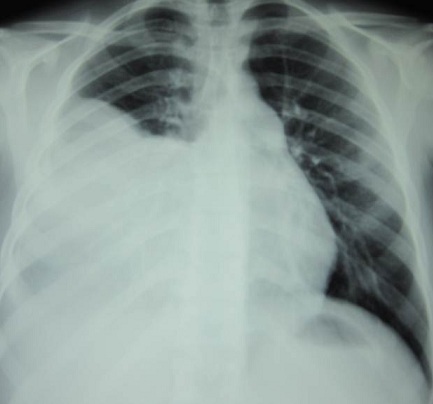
Chest radiograph showing a homogenous opacity in the right hemithorax
